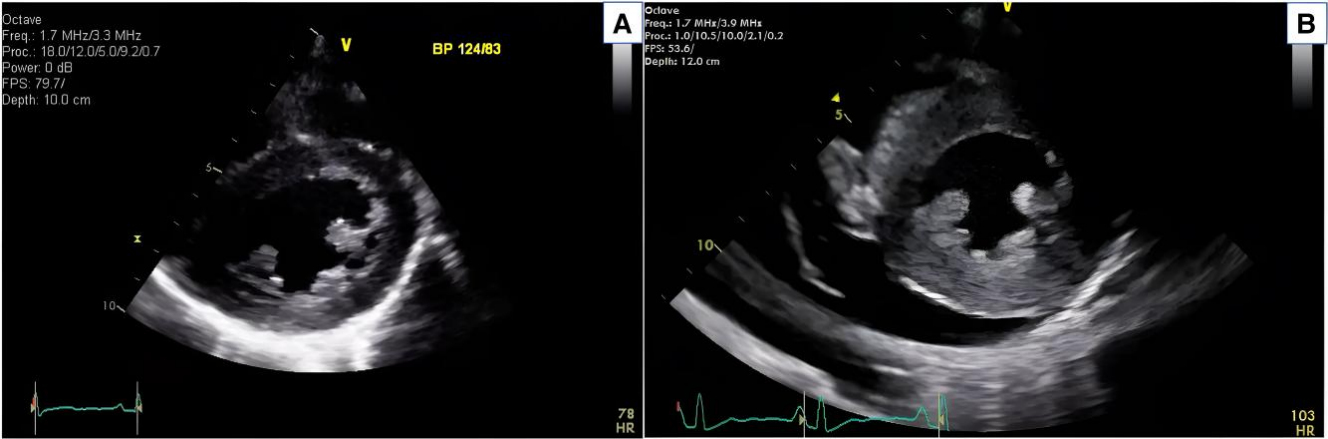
Echocardiogram showed *(A)* initial transthoracic echocardiogram (TTE), *(B)* follow-up TTE.
